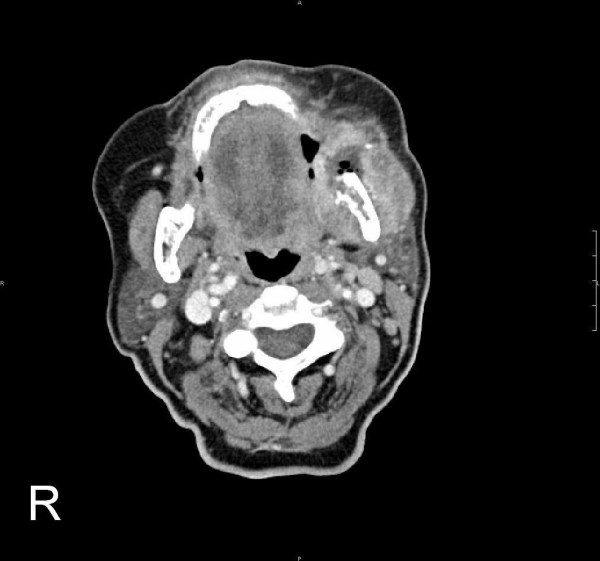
Computerized tomography (CT) with contrast medium (CM): Axial image of the head and neck: Tumour (4 × 5 cm^2^) of the left buccal soft tissues with central necrotic and partly calcified components and resorption of the left mandible.
